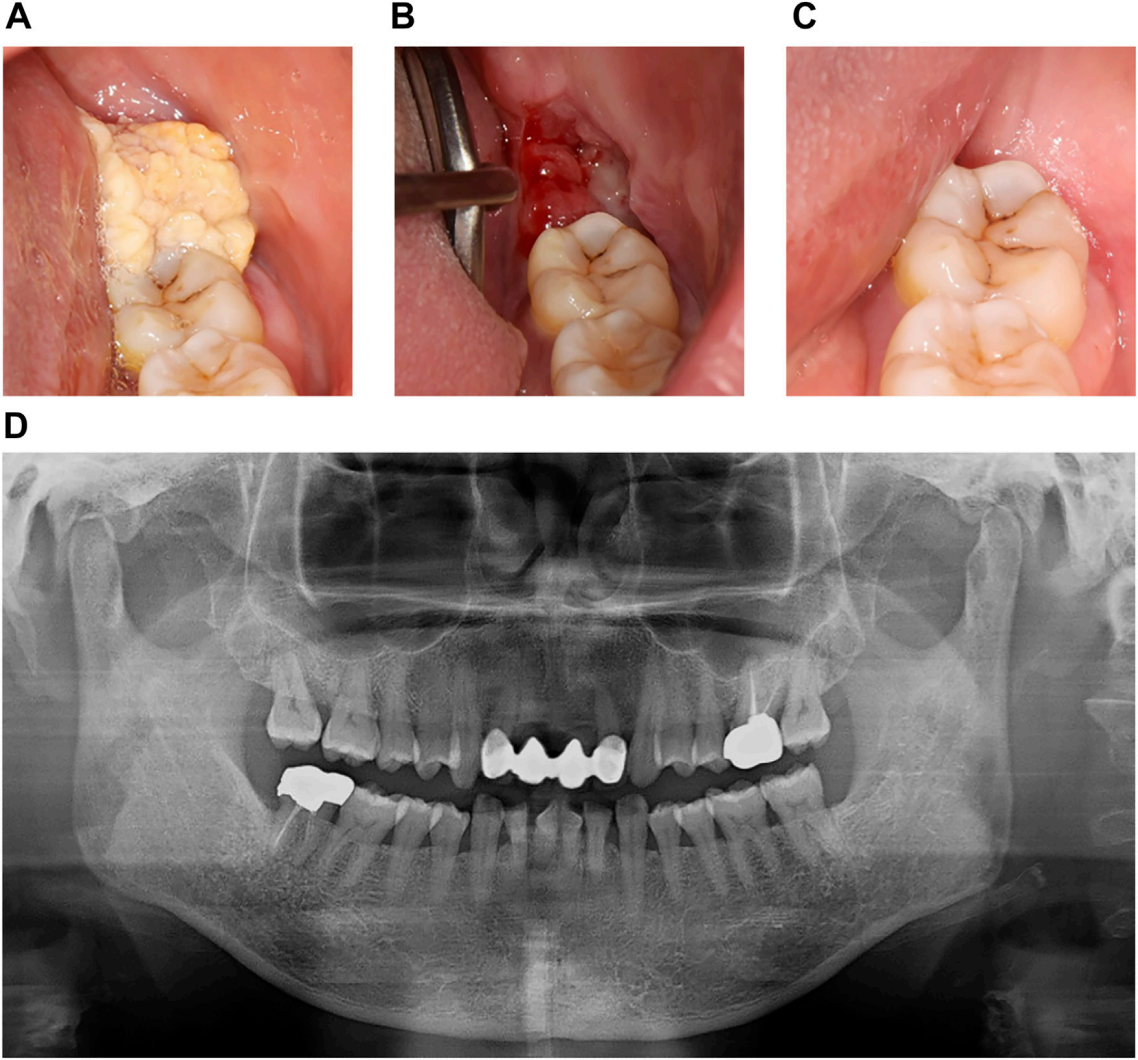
Clinical images and panoramic radiograph of the left mandibular lesion of case #1. (A) First visit. Verrucous exophytic, whitish-to-yellowish mass was observed on the left retromolar trigone area. (B) 4 weeks after the first visit and excisional biopsy. Papillomatous lesion, about 3 mm in diameter, recurred on the excision site. (C) 22 weeks after the first visit. There was no remarkable recurred lesion on the left retromolar trigone area. (D) Panoramic radiograph at first visit. Cortical erosion or bony destruction of mandible was not observed below the papillary lesion.
